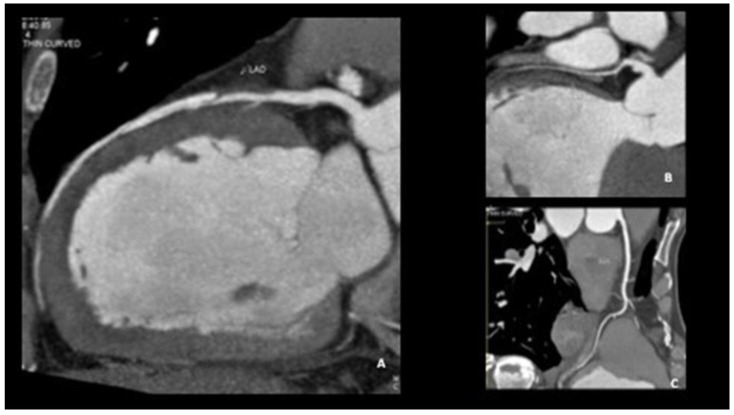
Coronary CT of a 42-year-old woman with STEMI, showing SCAD of the middle tract of LAD (A) and normal morphology of left circumflex (B) and right coronary artery (C). CT: computer tomography; STEMI: ST-elevation myocardial infarction; SCAD: spontaneous coronary artery dissection; LAD: left anterior descending.
